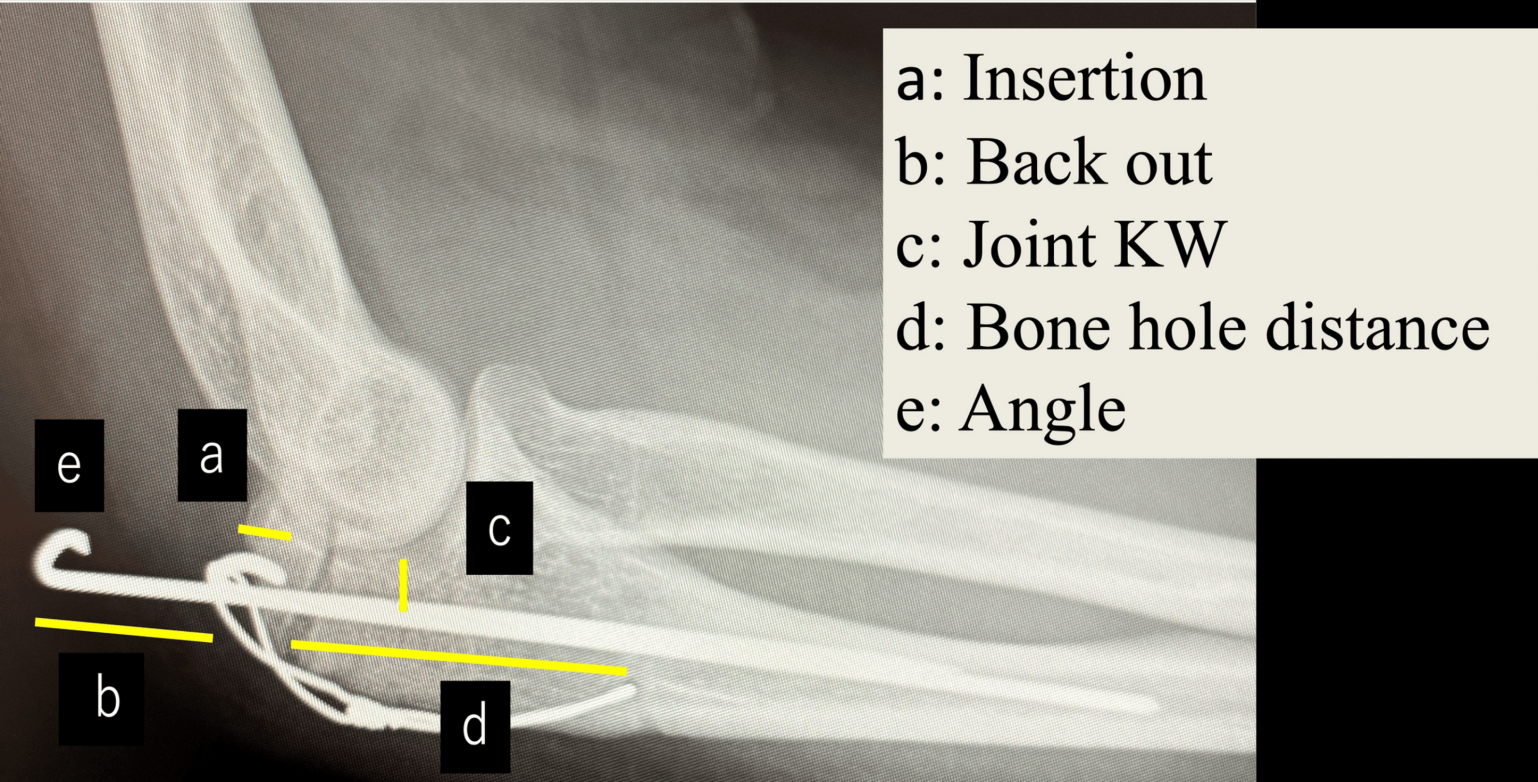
Measurement sites for each parameter in radiographs. The distances for each parameter were measured using X-ray images. (a) Length inserted into the bone at the bent end of the K-wire; (b) Backout distance, defined as the protrusion length measured from the dorsal cortical entry point of the olecranon to the bent proximal tip of the K-wire; (c) Distance from the olecranon joint surface to the K-wire; (d) Distance from the fracture line to the bone hole of the soft wire; (e) Bending angle of the K-wire end. K-wire: Kirschner wire
